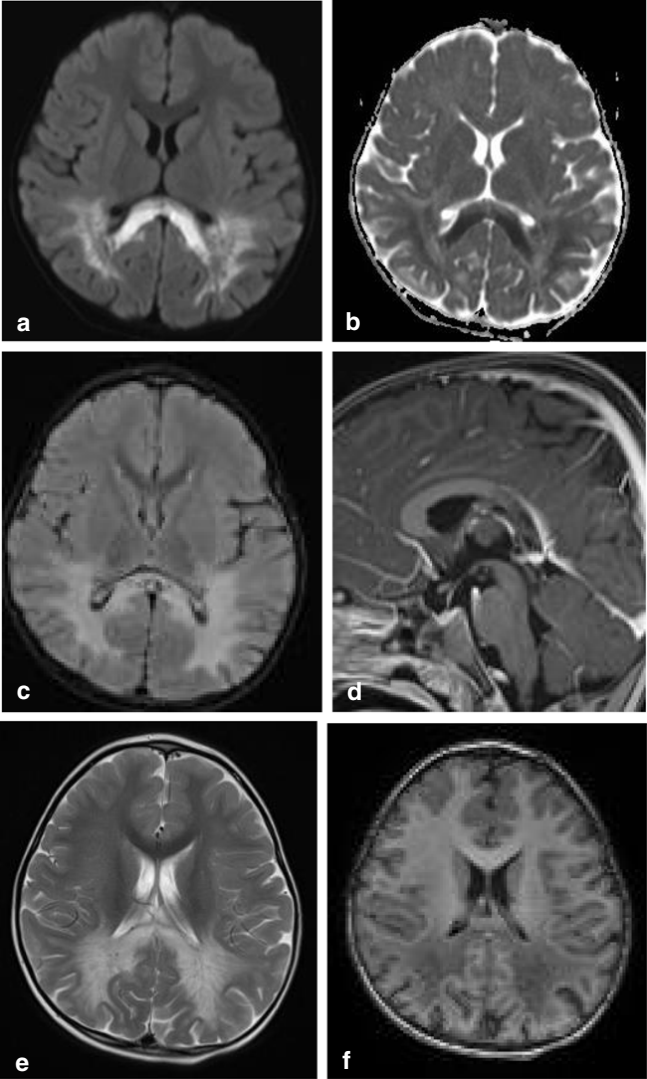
Three-year-old boy with Krabbe’s disease. Axial DWI (a) and ADC (b) show extensive abnormal signal with partial diffusion restriction involving the splenium and parietal white matter. Axial FLAIR (c) and sagittal T1 after gadolinium (d) show widened (hypointense) perivascular spaces in the splenium. Axial T2 (e) and inversion recovery (f) show spared axons in the parietal white matter
